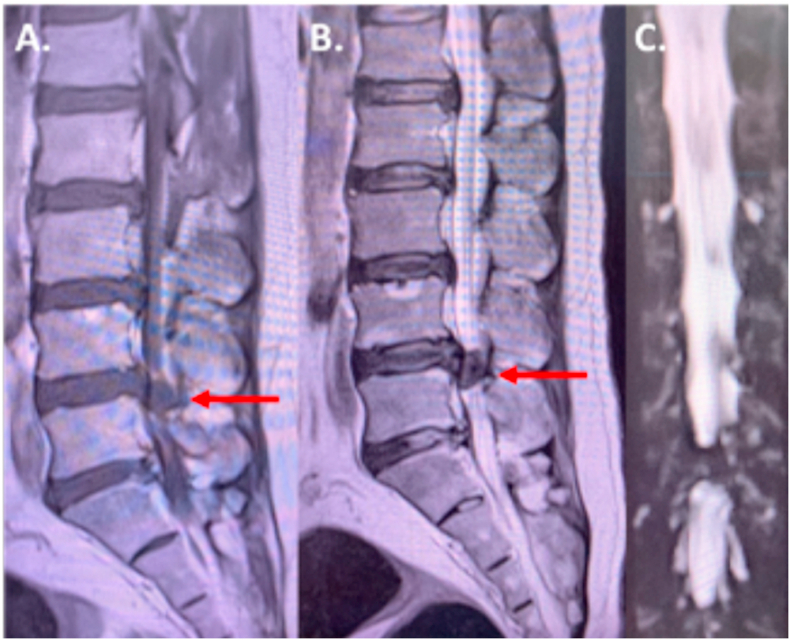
Sagittal section lumbar MRI showed a hypointense mass after gadolinium-injection on T1-weighted image (red arrow) (A) a heterogenous hypointense mass on T2-weighted image (red arrow) (B) and mass-like lesion on the MR myelography (C). (For interpretation of the references to colour in this figure legend, the reader is referred to the Web version of this article.)
